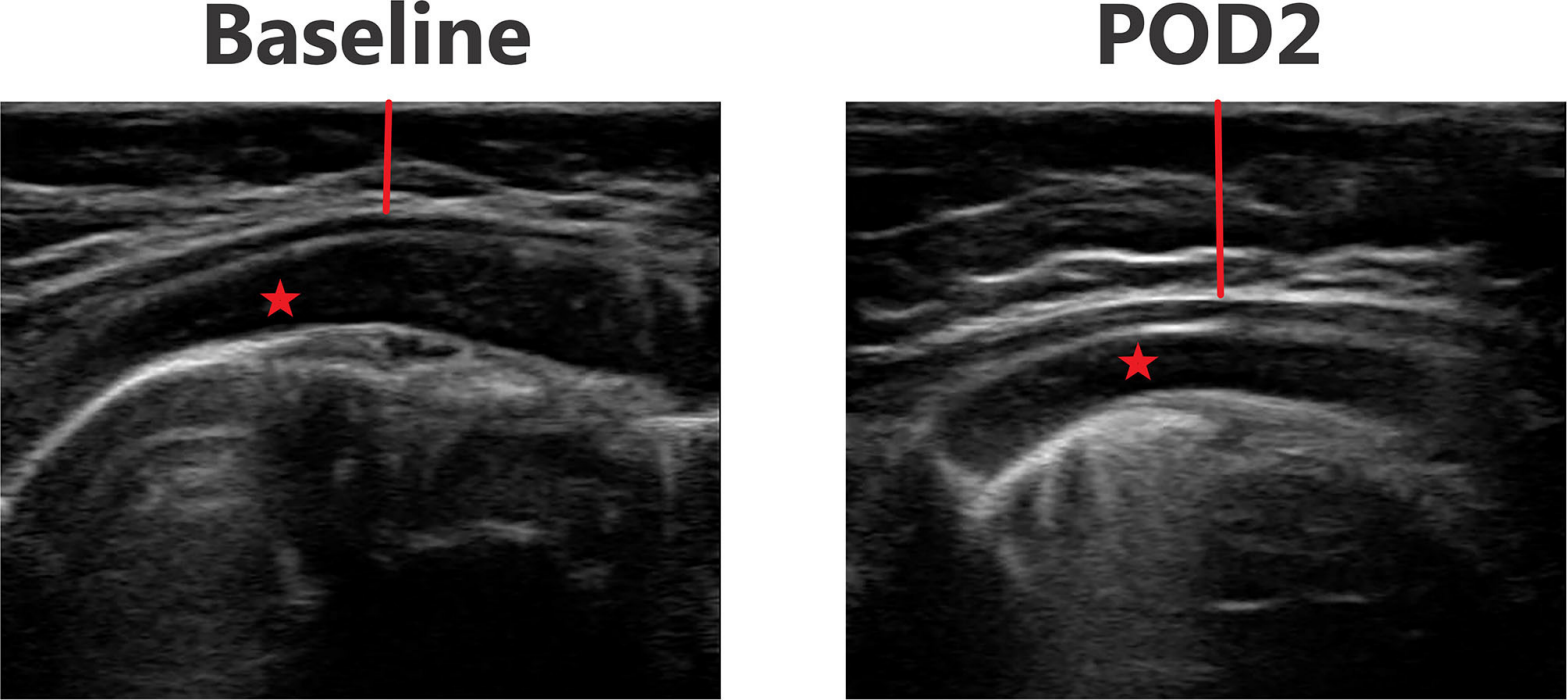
Point-of-care ultrasound images of anterior chest at baseline and POD 2 of the same patient. Both images are presented on identical scales (total depth of 2.5 cm from the skin). Red vertical line demonstrates subcutaneous tissue thickness—there is a marked increase in subcutaneous tissue seen on POD 2. Red star—pectoralis major muscle.
